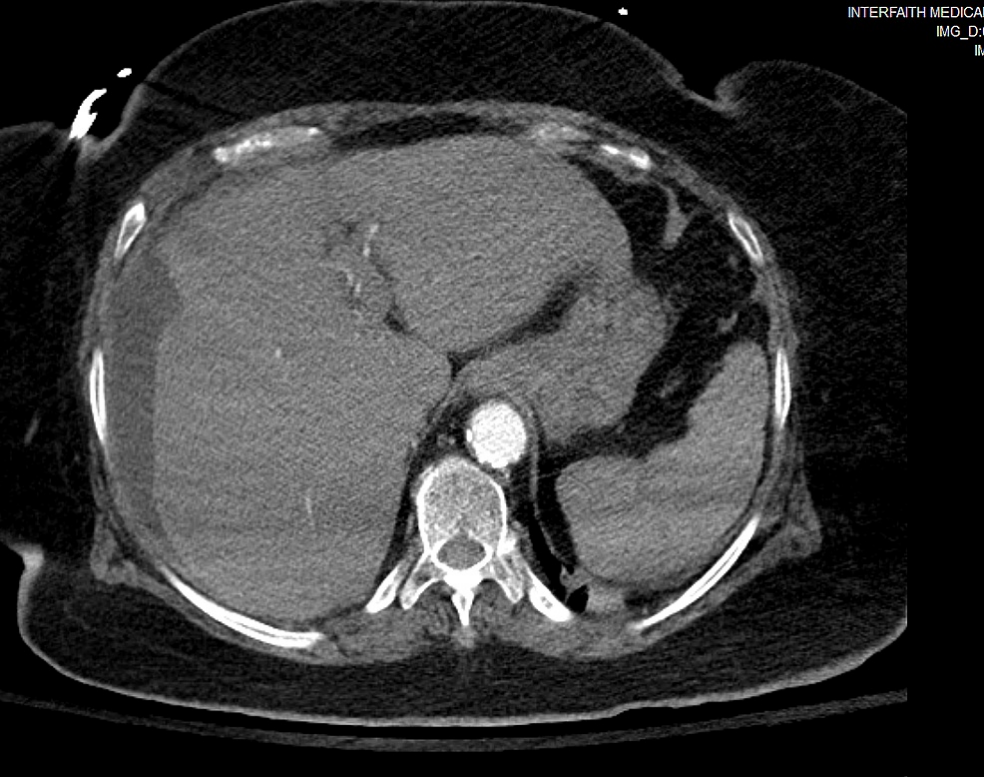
CT abdomen showing peri-hepatic fluid
